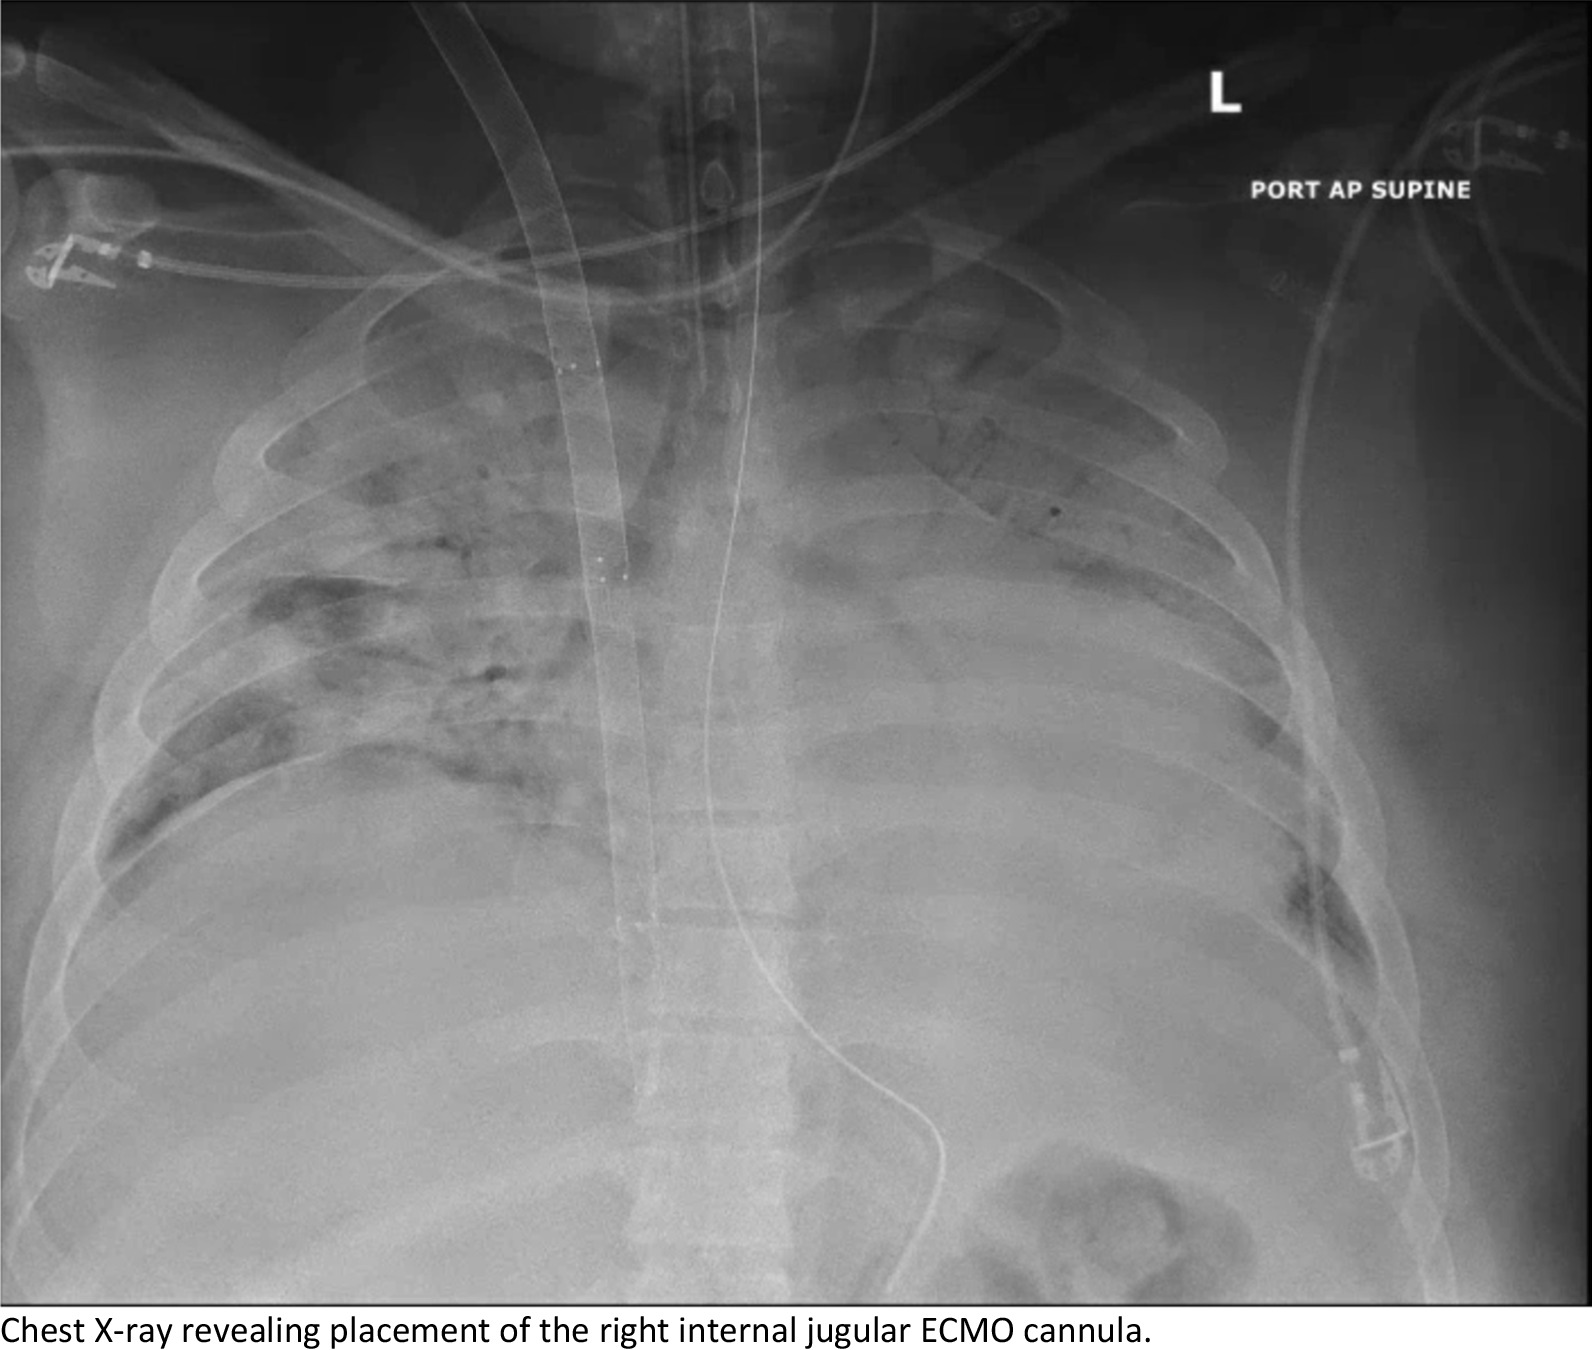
Chest X-ray revealing placement of the right internal jugular ECMO cannula
